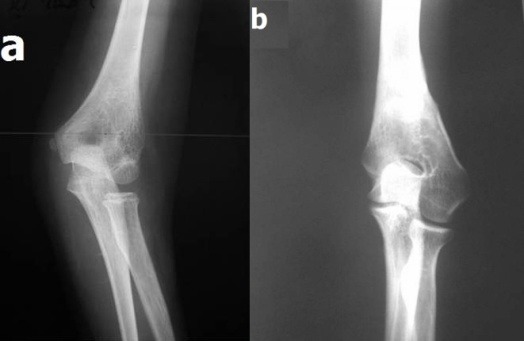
a. A marked deviation of the elbow that exceeds 40 degrees after a malunion of a supracondylar fracture of the humerus b. The axis of the thoracic limb is normal at growth end and the X-ray shows the osteoplastic effect of the intervention
